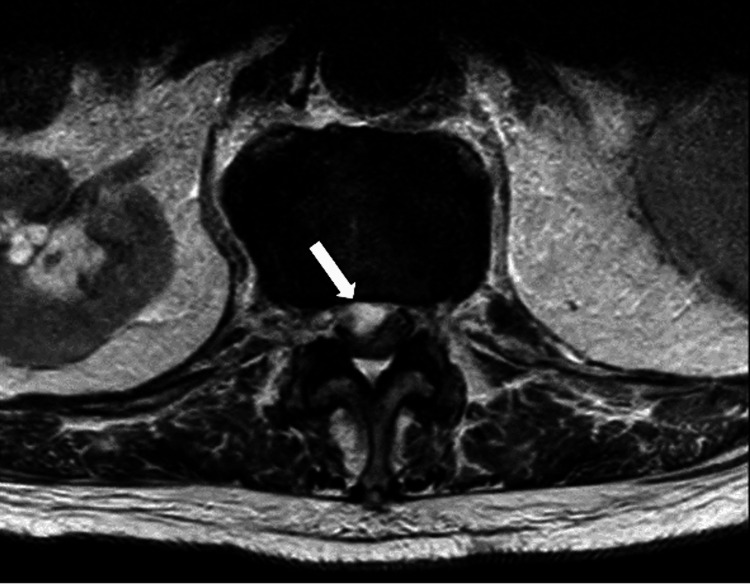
Lumbar MRI at presentation Transverse T2-weighted sequence showing high-degree spinal cord compression without cerebrospinal fluid (CSF) surrounding the spinal cord at level Th12/L1.
